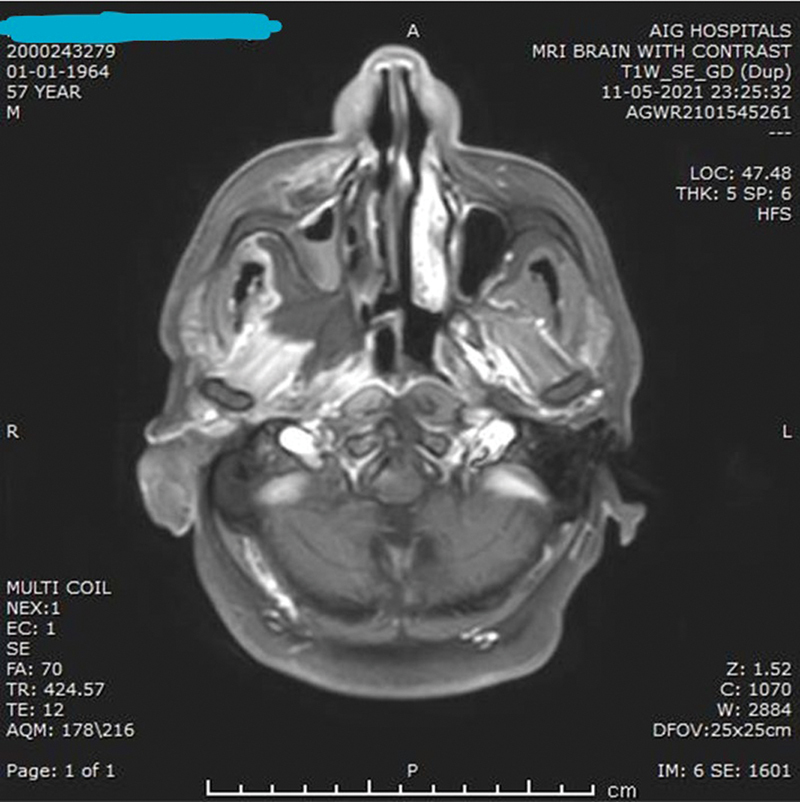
MRI OF BRAIN, PNS AND ORBITS 1. Sinusitis with necrosis involving the right maxillary, right ethmoid, bilateral frontal and sphenoid air cells. Extensive involvement of the right preantral, right retroantral soft tissue, the right masticator space, the right infratemporal fossa and the right palate with necrosis. Right-sided mastoiditis. 2. Changes of right-sided orbital and periorbital cellulitis with changes of optic neuritis,partially necrotic extraocular muscles with soft tissue in the right orbital apex and cavernous sinusFindings are in keeping with extensive rhio-orbital mucormycocis.
